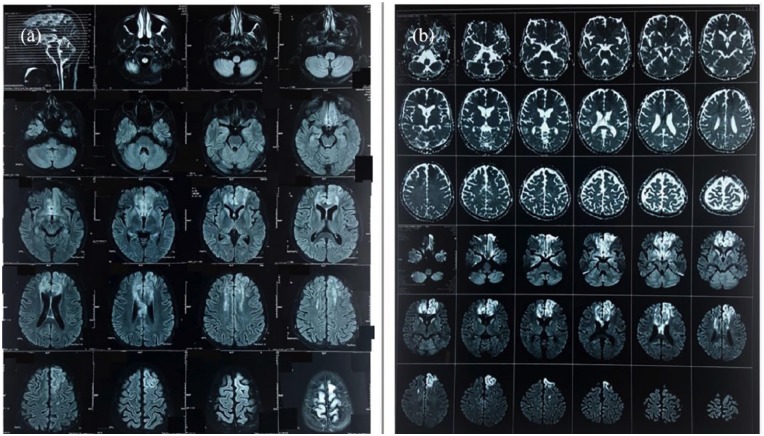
(a) MRI FLAIR axial images showing cortical and subcortical white matter
